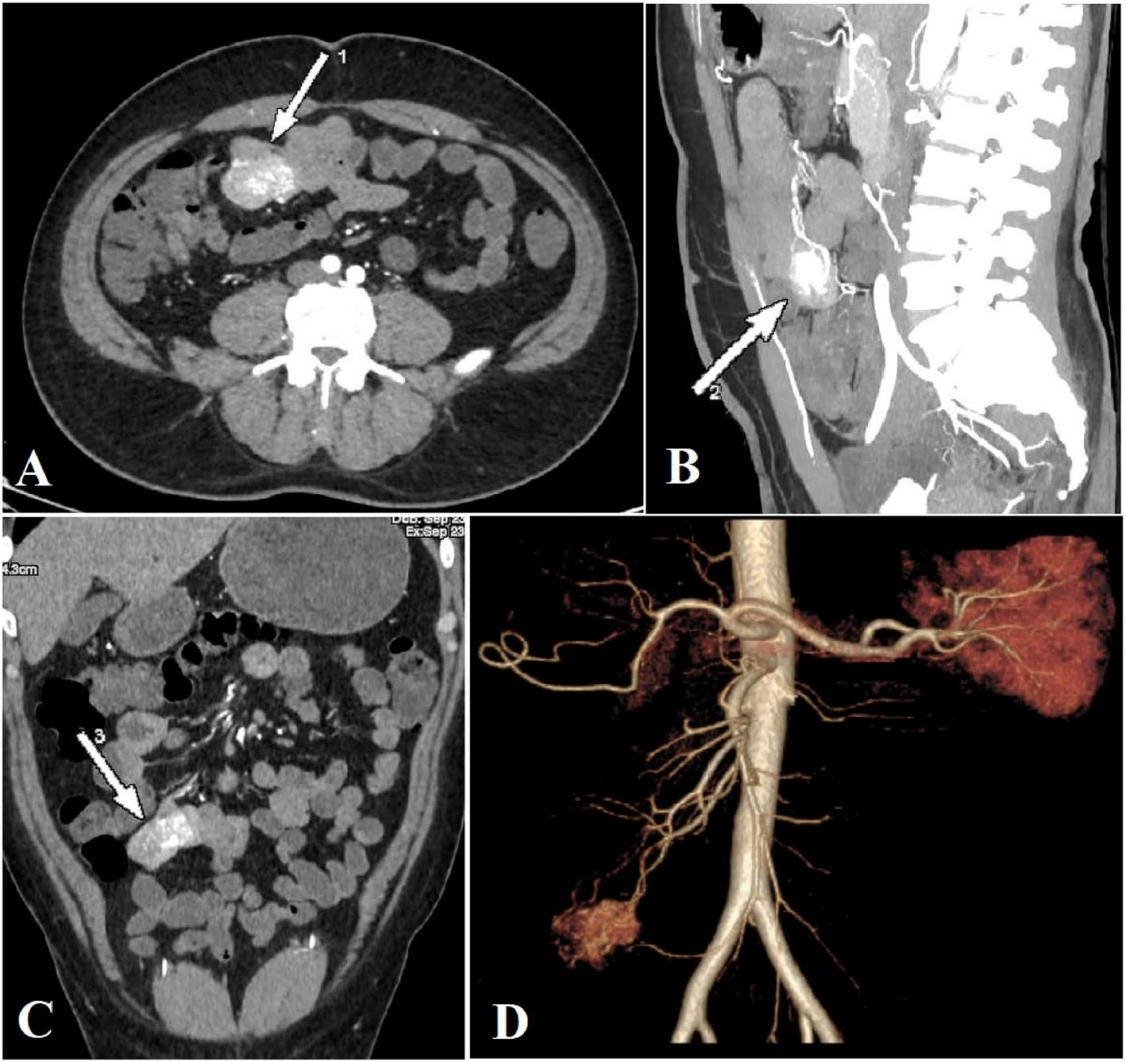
A, B and C Triphasic pelviabdominal cross sectional, sagittal and coronal CT angiogram Reviewer (2), views showing a hypervascular ileal GIST. D 3D reconstruction of the CT angiogram Reviewer (2), showing that the mass supplied from the ileocolic artery
